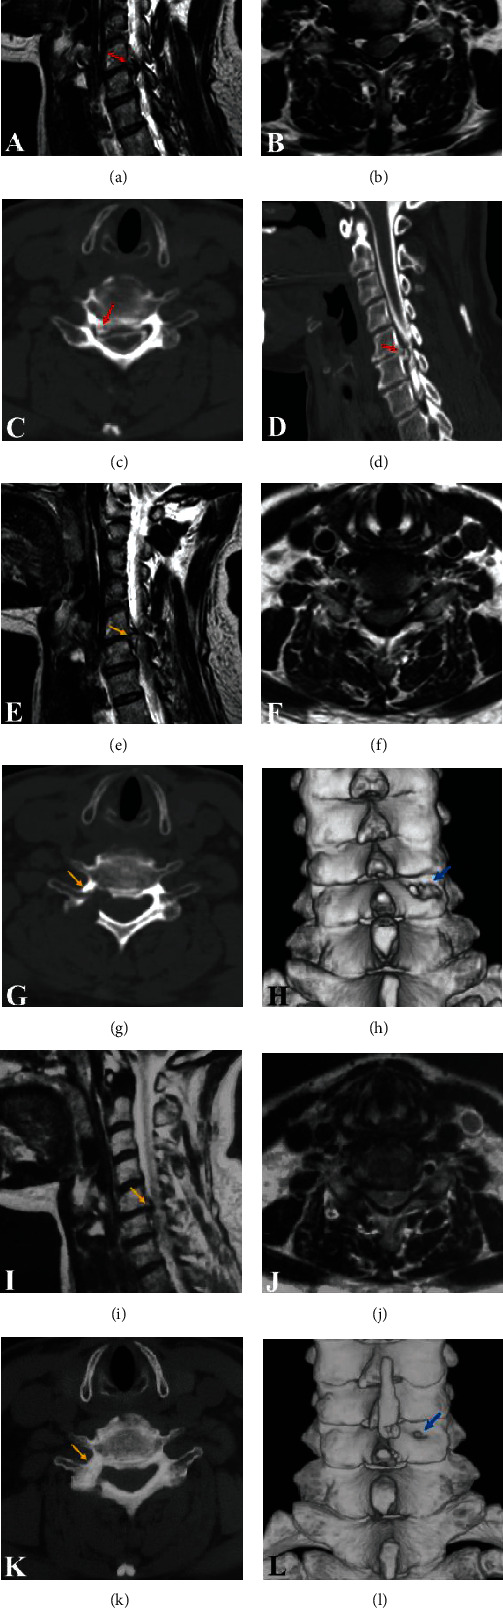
(a) Sagittal MRI scan showing a severe compression of the C5-C6 cervical segment. (b) Axial T2-weighted MRI of the C5-C6 cervical segment. (c) Preoperative CT myelography image showing an osteophyte compressing the lateral portion of the spinal cord. (d) Reconstructed parasagittal CT myelography image showing compression of a mixed type. (e, f) Postoperative sagittal and axial MRI scan showing total decompression. (g) Postoperative CT scan showing partial removal of the lateral mass and pedicle and removal of the osteophyte. (h) 3D reconstruction showing a hole in the lateral mass. Note that the facet joint is intact. (i, j) Postoperative sagittal and axial MRI scans showing no herniated disc at 12 months postoperatively. (k) CT scan at 12 months postoperatively showing that the pedicle and lateral mass defects are obviously decreased. (l) 3D reconstruction showing that the hole in the lateral mass is dramatically decreased compared with its size immediately postoperatively.
